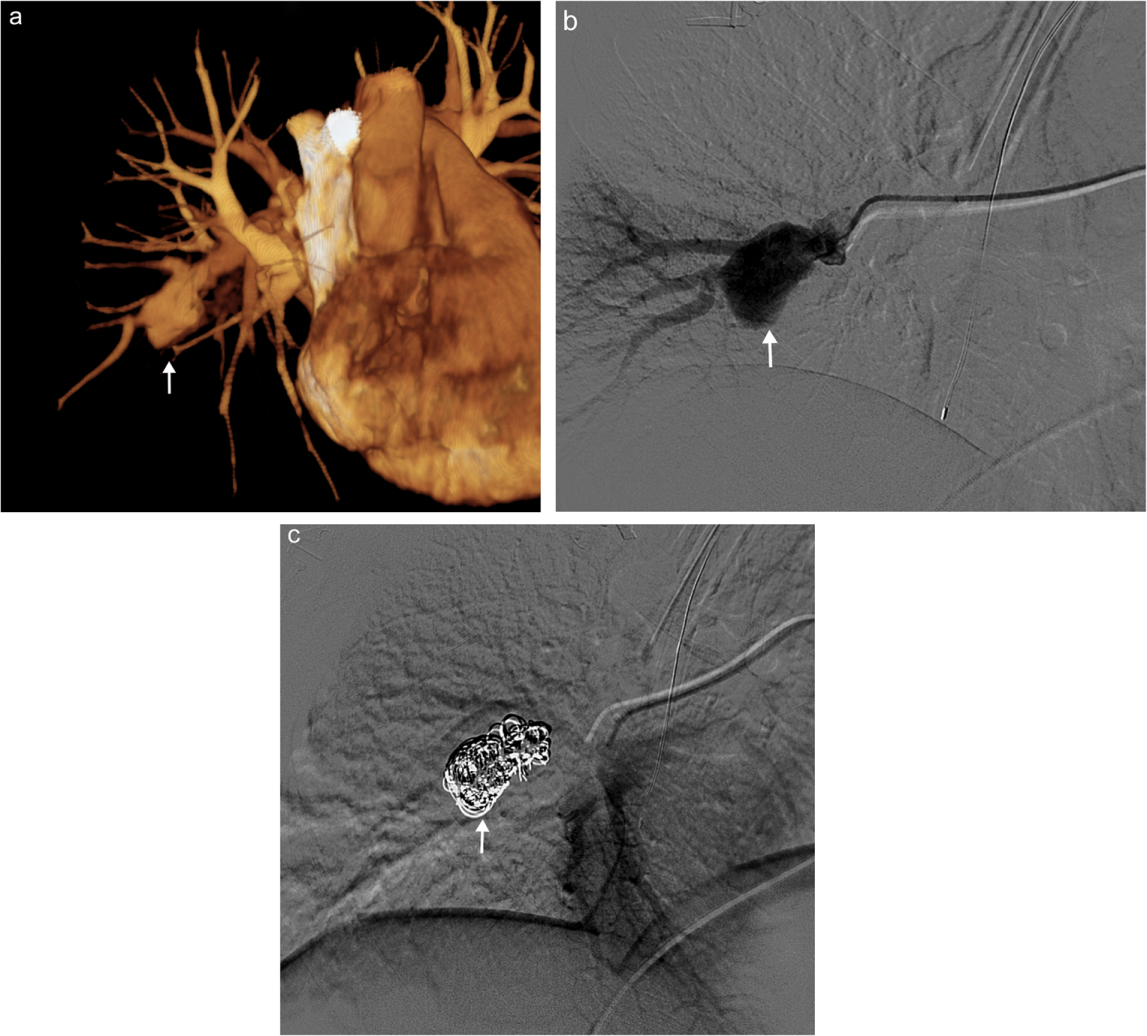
Pulmonary artery aneurysm in a patient with Behçet’s disease. a Three-dimensional reconstruction from a contrast-enhanced CT scan showing a right lower lobe pulmonary artery aneurysm in a patient with Behçet’s disease (*white arrow*). Digital subtraction fluoroscopic images obtained before (b) and after (c) coil embolisation of the right lower lobe pulmonary aneurysm (*white arrow*)
